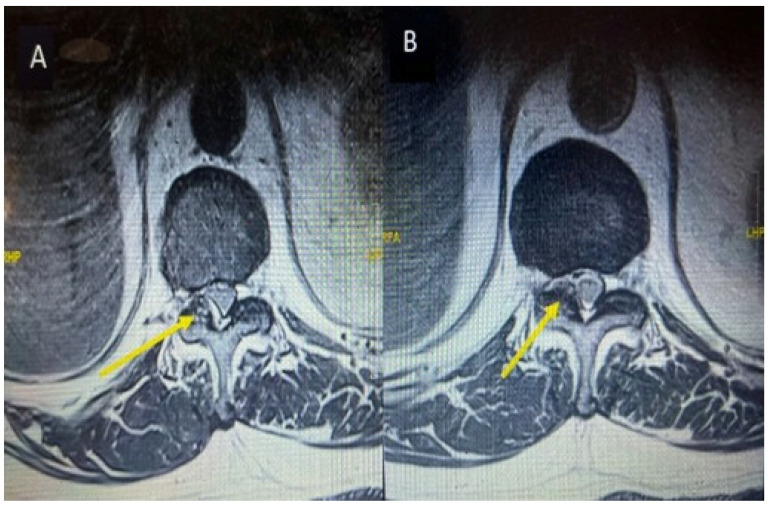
MRI thoracic spine T2-weighted at the level of T10/11 axial section before starting anti-gout medications (A) and axial section of follow-up MRI after starting anti-gout medications (B) showing a cyst on the right side as indicated by yellow arrow and total disappearance as shown by yellow arrow after starting anti-gout medications.
